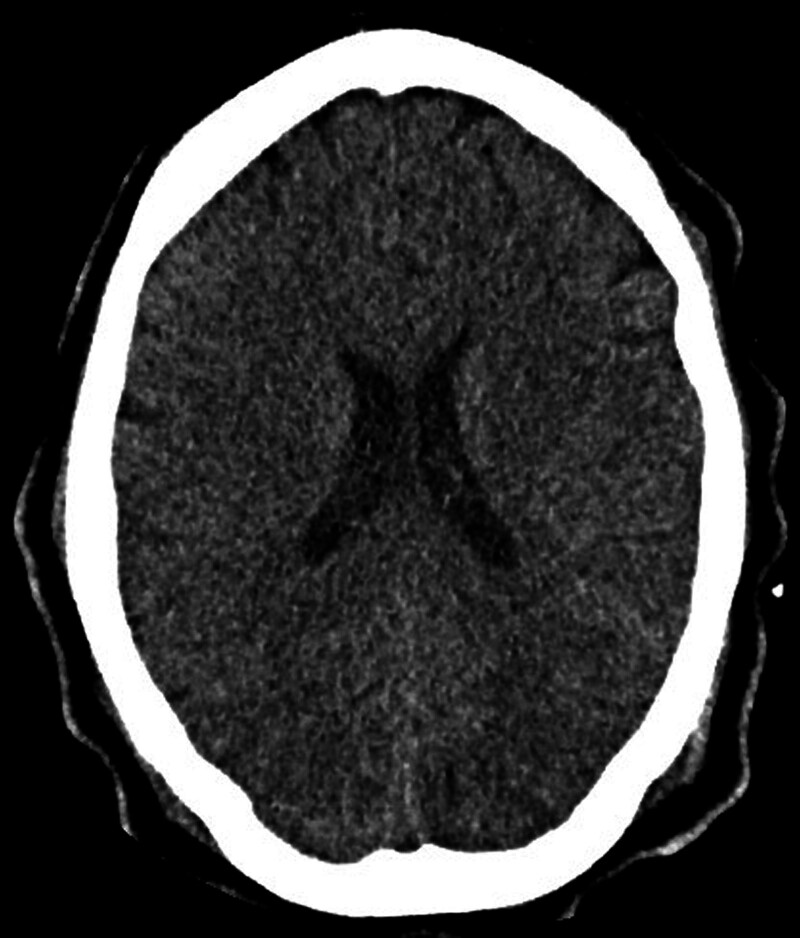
Non-contrast cranial CT after status epilepticus. CT = computed tomography.
